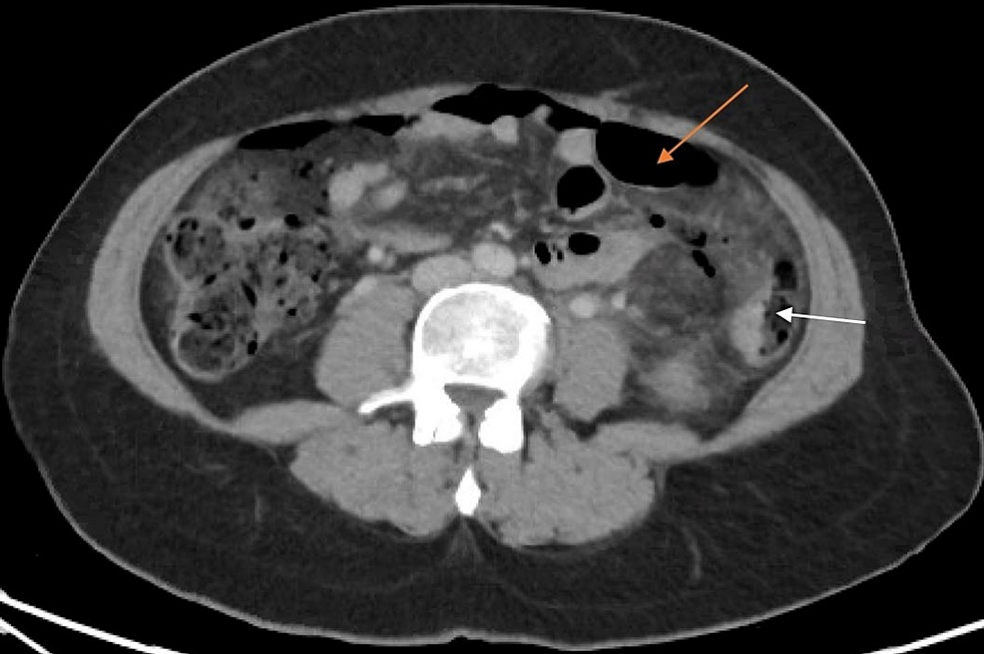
CT abdomen/pelvis without contrast (axial image) showing a moderate amount of pneumoperitoneum (orange arrow) with diverticulosis present (white arrow) in the mid-descending colon
